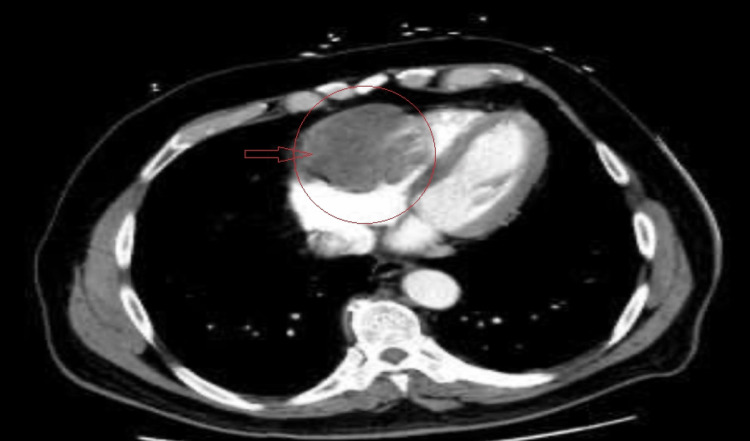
The chest CT scan shows a right ventricular mass
